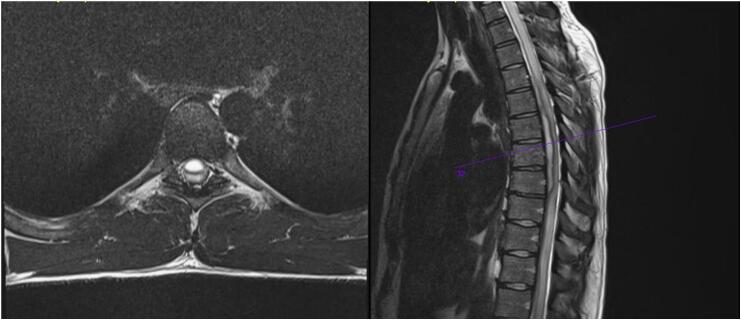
Axial/sagittal T2 MRI demonstrating ventral abscess. Mass appeared to be epidural and measured at least 6 mm × 10 cm in length. Gross distortion of the spinal cord with local edema as well as menial enhancement through the thoracic spine can be seen.
